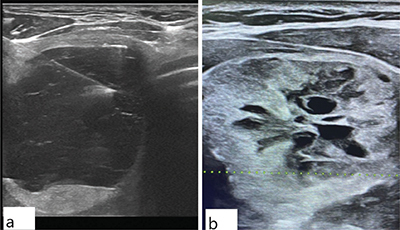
Ultrasound images of thyroid nodules with different categorizations. (a) This image depicts a nodule with cystic features, characterized by smooth margins and floating colloid, along with echogenic foci and debris (TI-RADS 1). The fine-needle aspiration cytology (FNAC) results for this nodule indicated a nondiagnostic (ND) outcome. (b) It exhibits a solid composition, an iso-hypoechoic appearance, a heterogeneous texture, central cystic regions, and a lobulated margin (TI-RADS 4). The FNAC results for this nodule indicated atypia of undetermined significance (AUS).
